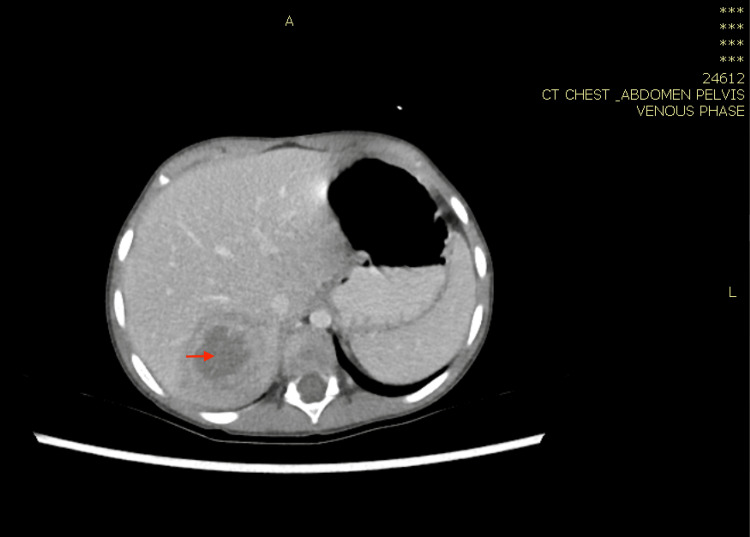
Computed tomography of the chest showing fairly well defined peripherally enhanced, centrally hypoattenuating lesion around 45 Hu in the 6th to 7th liver segments with a thick, irregular wall measuring 3.8 cm × 4 cm × 2.8 cm associated with surrounding peripheral edema (red arrow).
